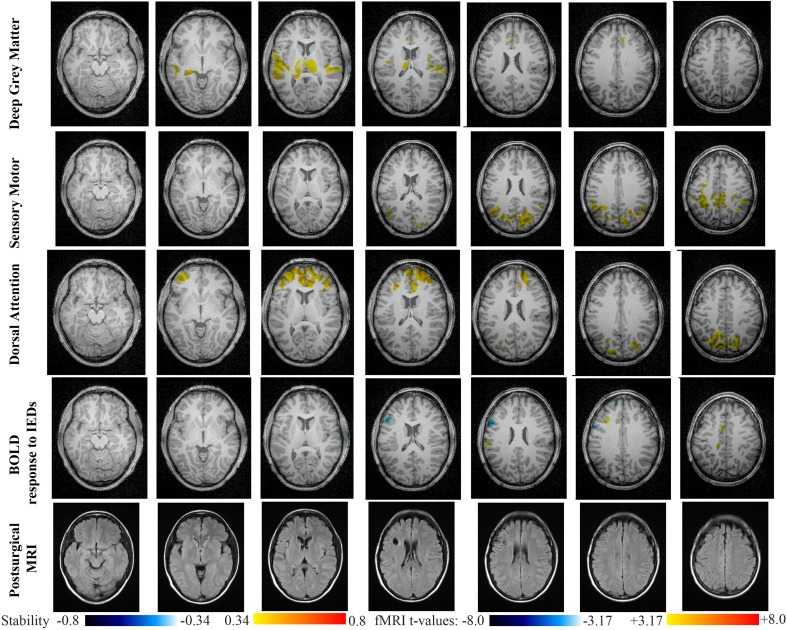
Evaluation of DANI on Patient 5 with left frontal FCD. Results are presenting most salient stability changes observed in *Cmap*_*n*_ followed by a *t*-map of the BOLD response to epileptic discharges and a postsurgical FLAIR MRI, all resampled in the native anatomical MRI space of the patient. Most salient stability changes observed for *Cmap*_*n*_ of Deep Gray Matter, Sensory Motor, and Dorsal Attention networks are presented. No networks were involved in significant interactions of modularity changes at *p* < 0.001. Same colormap conventions than in Figure 6.
